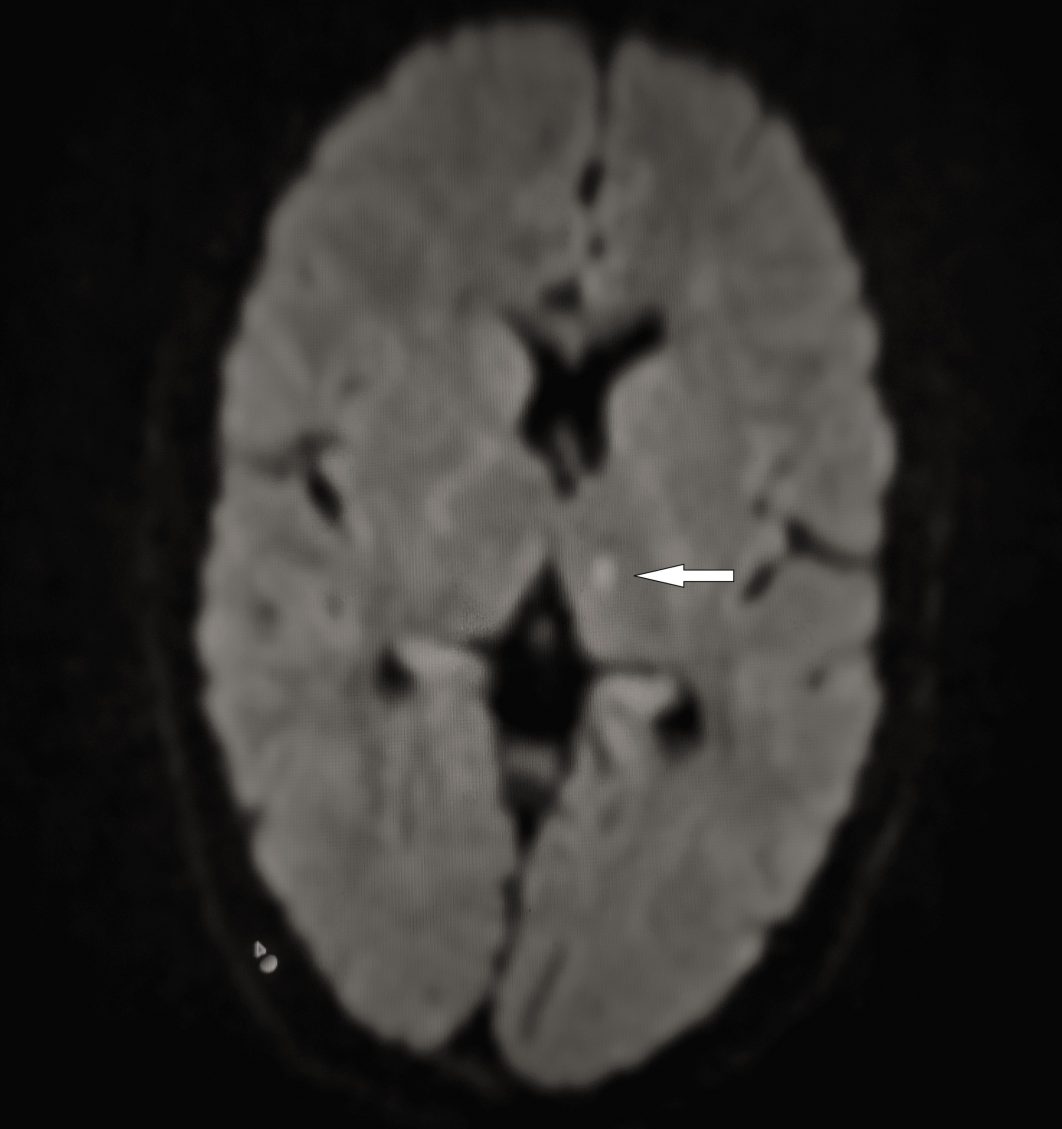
Diffusion-weighted MRI of the brain demonstrating a hyperintense area of restriction in the medial thalamus
